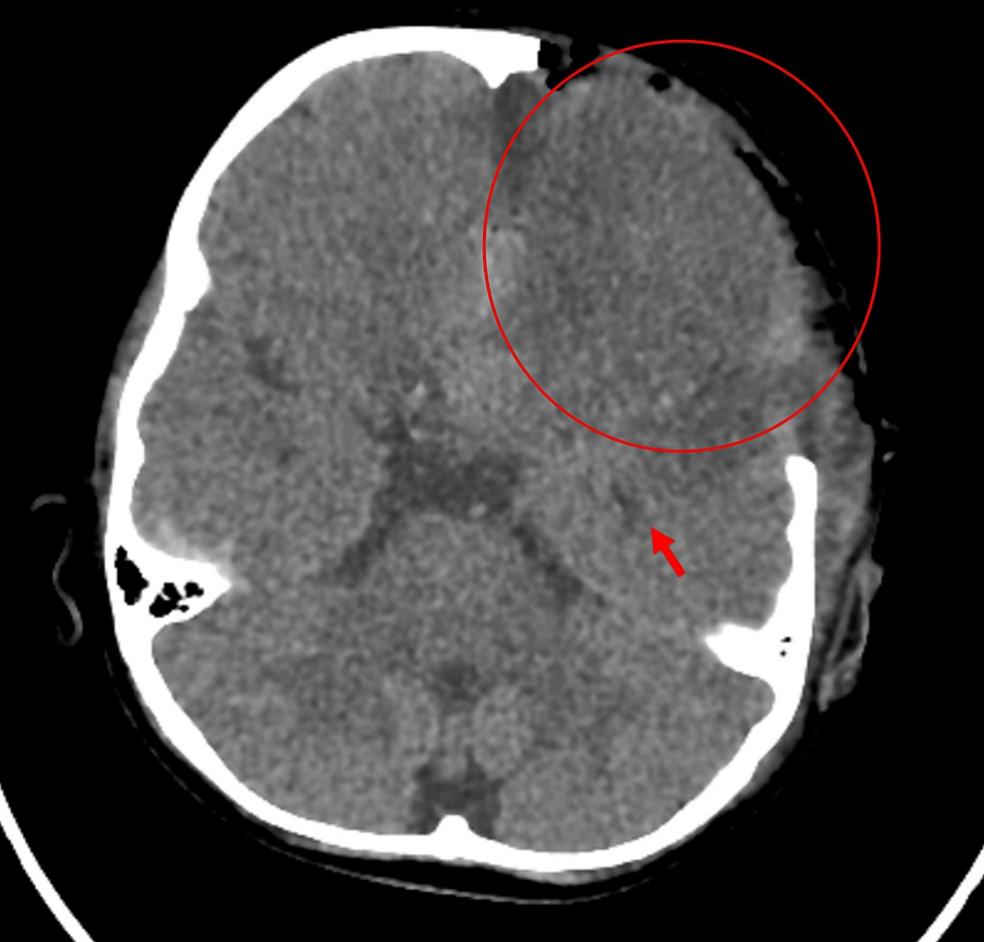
CT of the brain Left hemisphere edematous changes with midline shift S/P left frontoparietal decompression craniectomy (red circle). Effacement of the left lateral ventricle (red arrow). CT: computed tomography, S/P: status post
